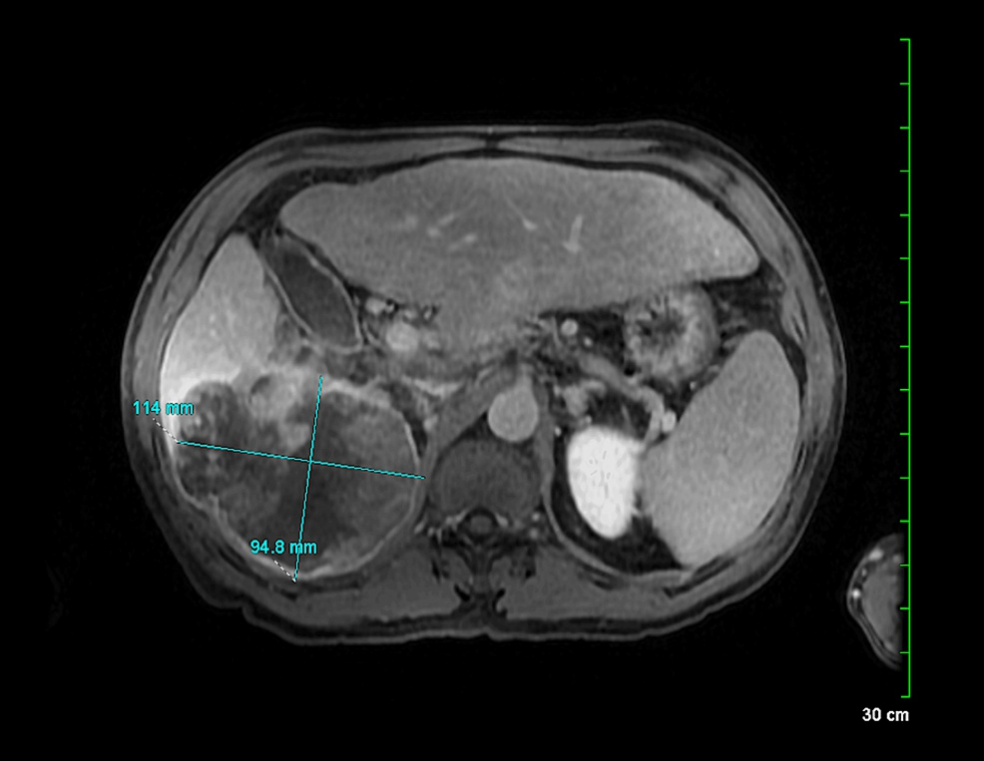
T1 MRI Liver Mass Protocol Magnetic resonance imaging shows a lobulated heterogeneously enhancing mass within the posterior and inferior right hepatic lobe abutting the right kidney.
